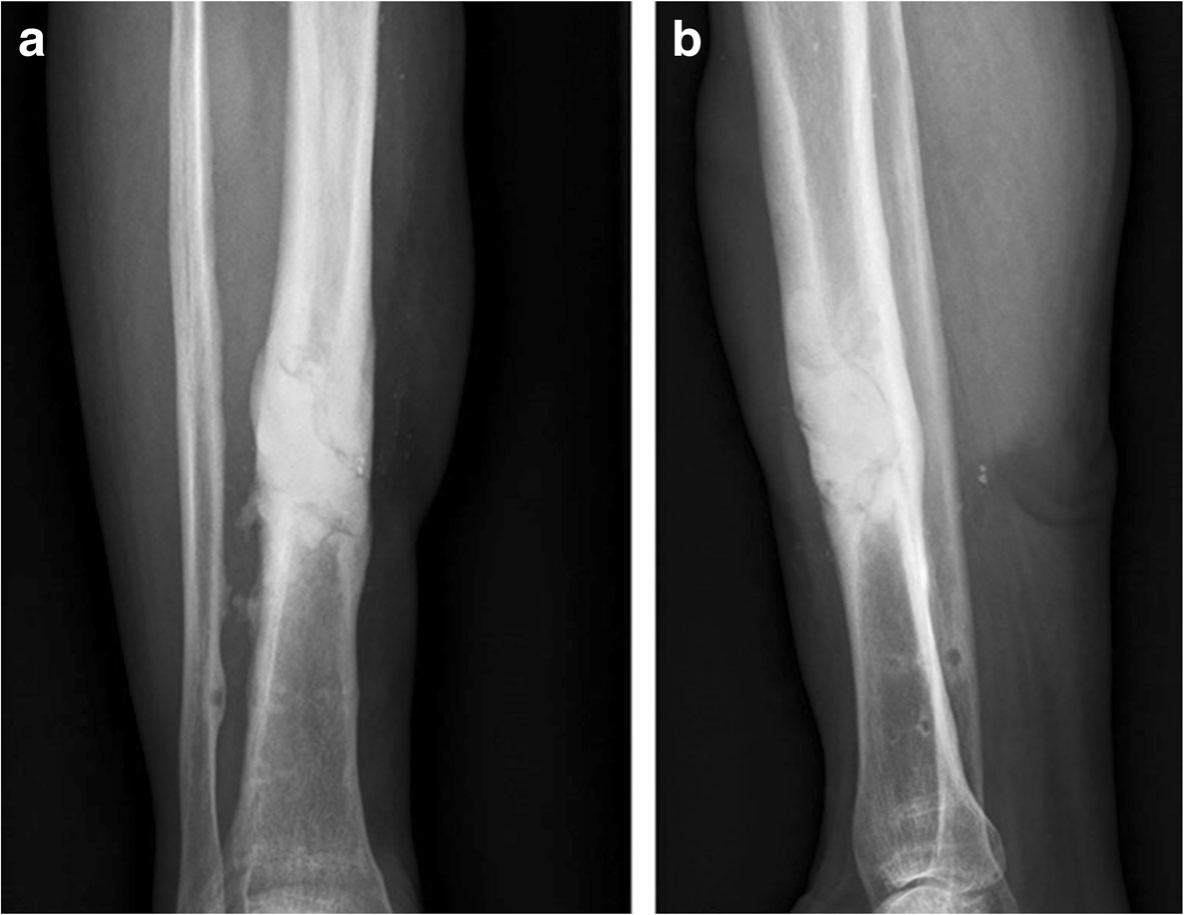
Postoperative radiographs showed that PMMA spacer was absorbed filled in the bone cavity after surgical treatment. (a) posteroanterior, (b) lateral
